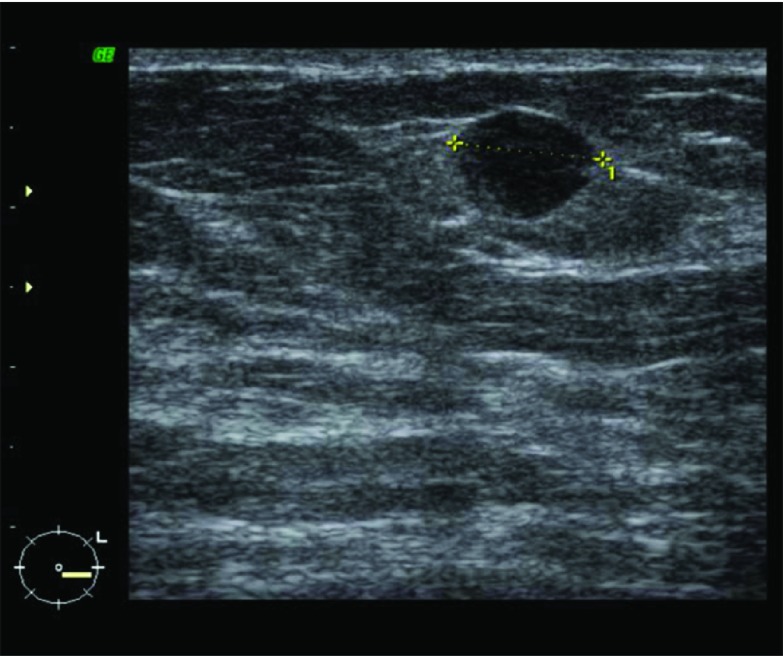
Breast ultrasound demonstrates a 9-mm predominantly well-defined hypoechoic mass in the outer half of the left breast corresponding to the mammographic abnormality.
